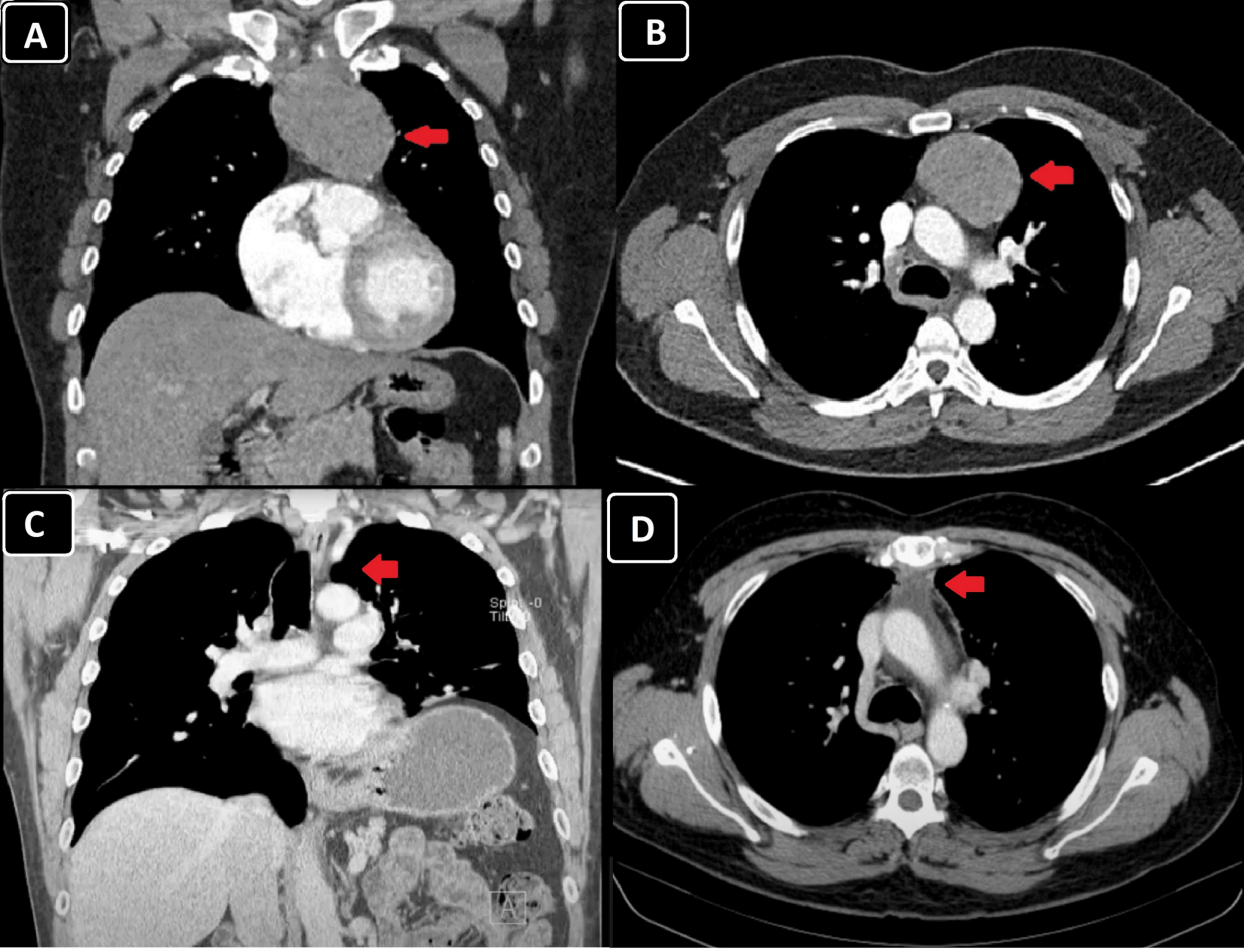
Computed tomography of the chest, in both (A) the coronal and (B) the axial planes, reveals an oval nodular lesion (red arrow) measuring approximately 7 cm in the longest axis and 4.5 cm in the shortest axis (on the axial plane), located in the anterior mediastinum. The lesion demonstrates heterogeneous density following the administration of contrast. The images C and D show the resolution of the thymoma.
